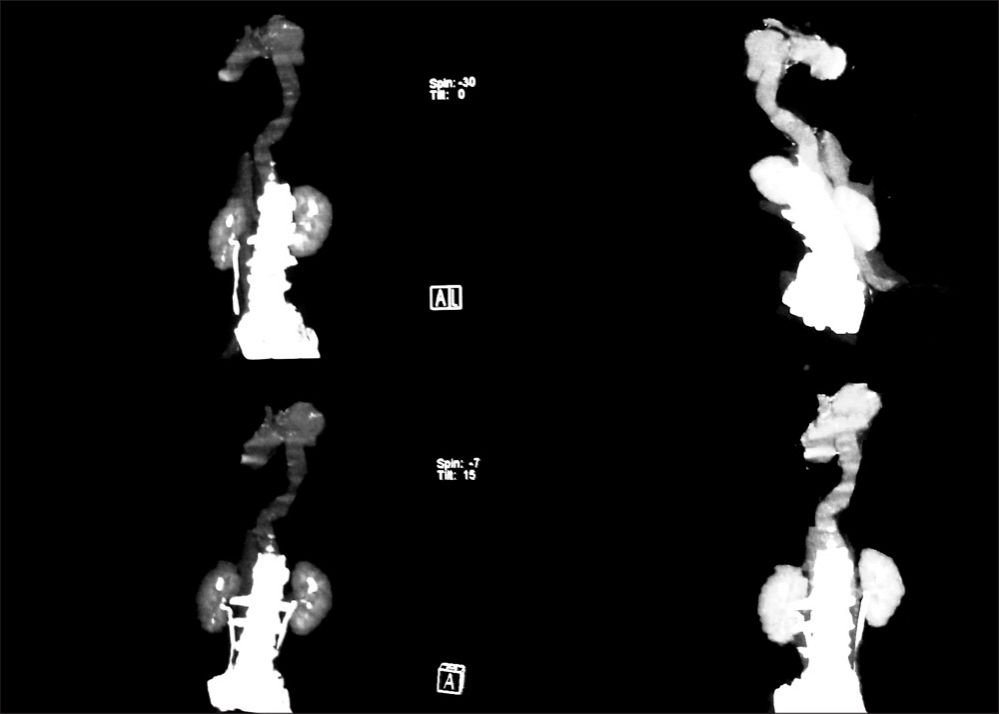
CT aortogram shows aneurysm of arch and descending aorta
